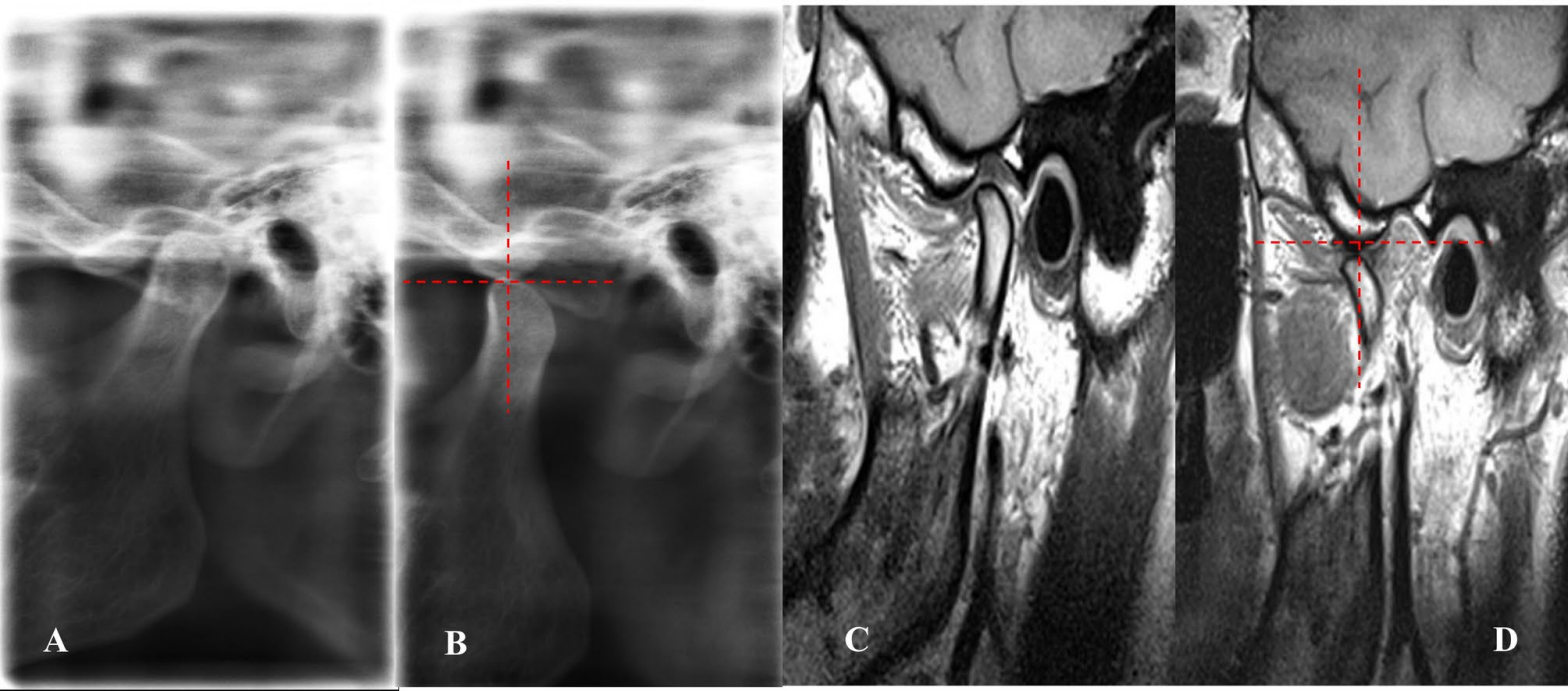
Lateral TMJ view representing Stage 0. Closed mouth (A) and open mouth (B) positions show the condyle located directly below the tip of the eminence. Oblique sagittal T1-weighted images representing Stage 0 in closed mouth (C) and open mouth (D) position show a normal condyle-disk-fossa relationship. The disk-condyle complex is located directly below the tip of the eminence
